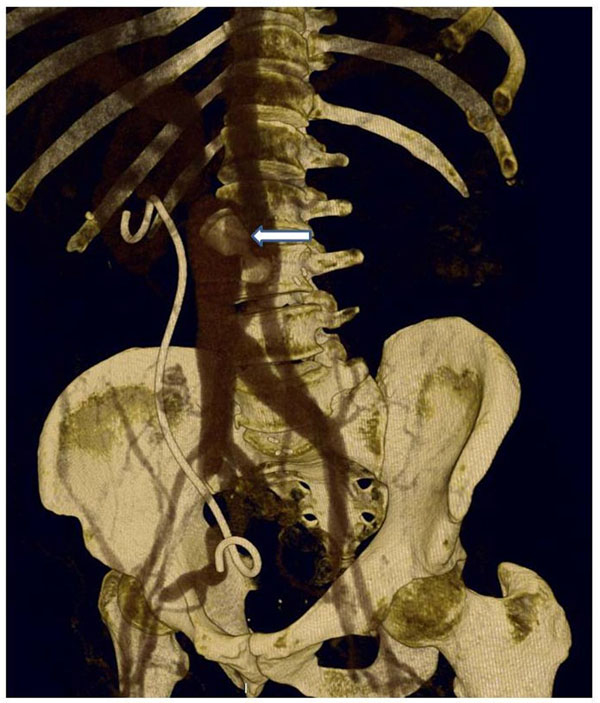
The CT scan at 1 month follow-up after vascular plug implantation showed recurrence of the aorto-caval communication. The Amplatzer vascular plug, still in correct position, was not able to halt the fistulous communication.
